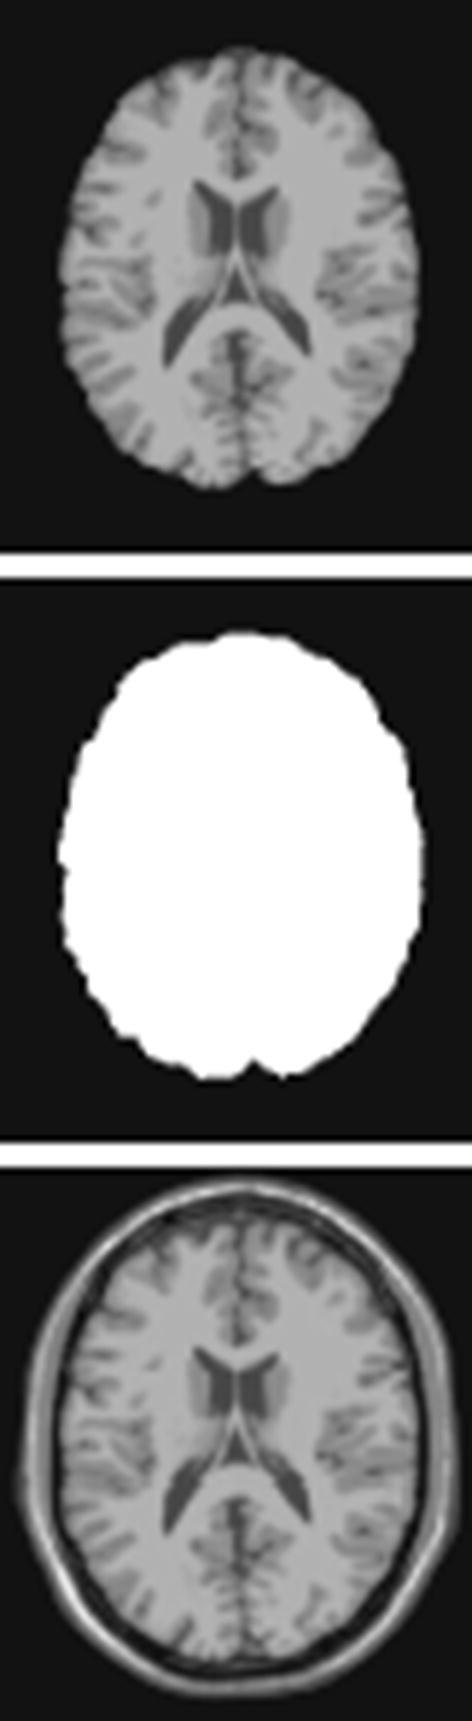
The MR image (top), its binary mask (middle) and the extracted brain region (down)
